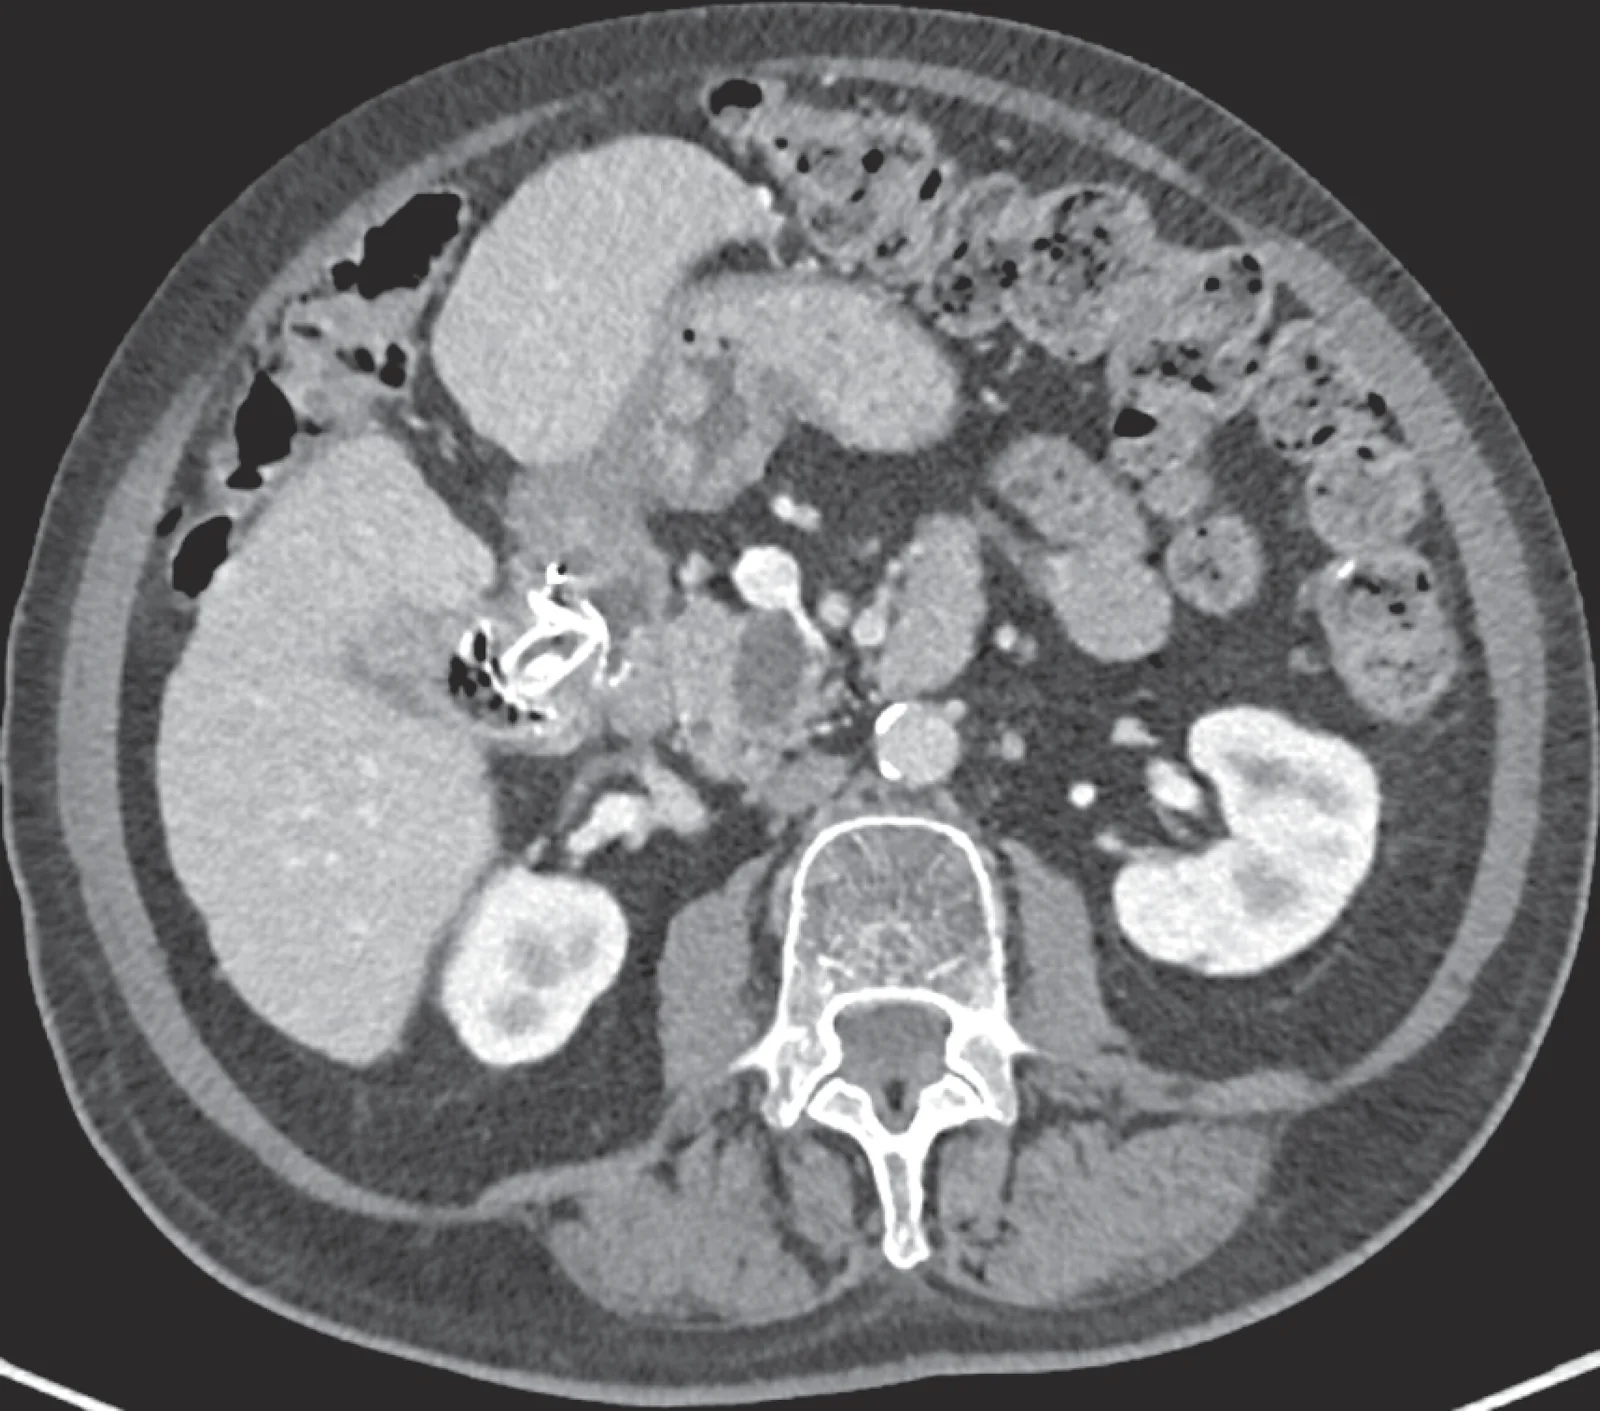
A CT scan showing the LAMS (Hot-SPAXUS, Taewoong, Gimpo, Korea)
